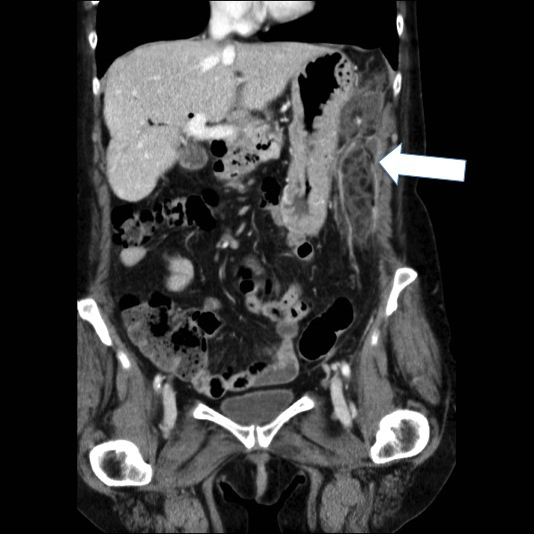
Coronal portal venous phase contrast-enhanced CT: arrow shows left upper quadrant fat density mass with peripheral enhancement adjacent to the greater curve of the stomach.
